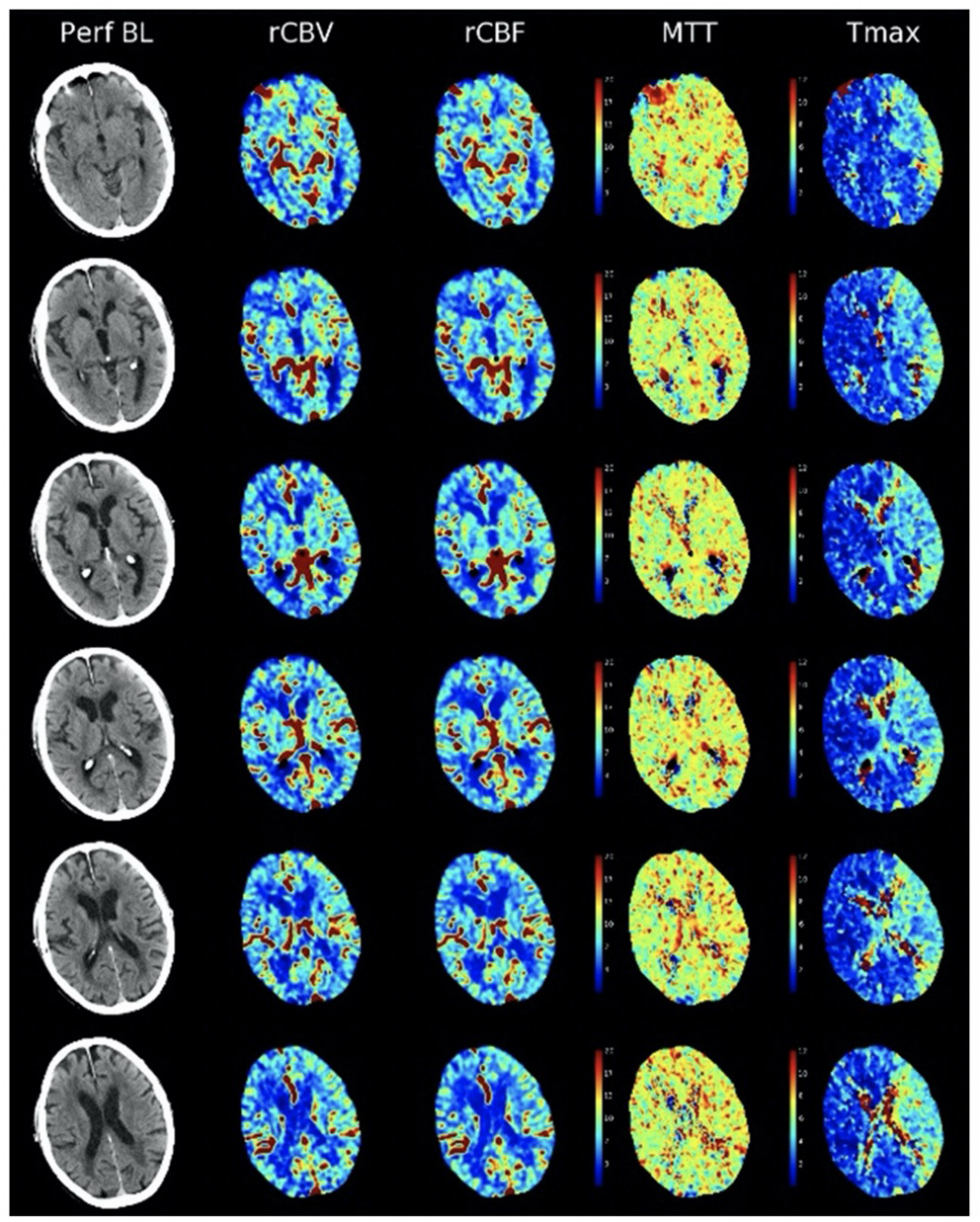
Non-contrast CT and CT perfusion maps of ICAD in the proximal left middle cerebral artery. A small, subcortical stroke is seen on non-contrast CT (left column, Perf BL). CT perfusion (right columns) reveals residual antegrade flow across the stenotic lesion, with preserved blood volume (rCBV), blood flow (rCBF), and mean transit time (MTT) with extensive areas of Tmax delays <6 s (Tmax).
